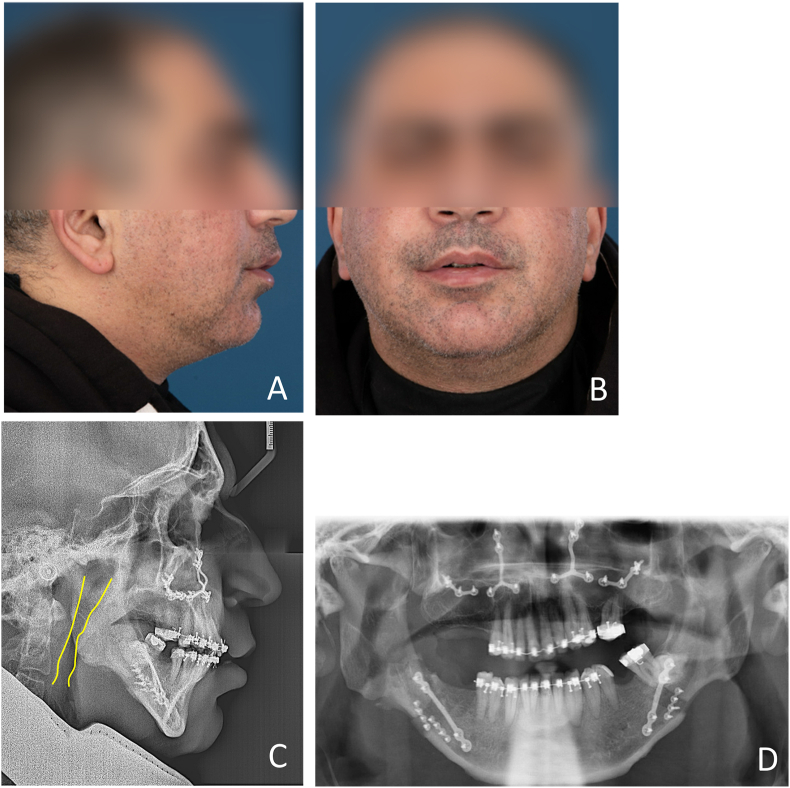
After maxillomandibular advancement surgery. A, profile view of the extra-oral photograph; B, frontal view of the extra-oral photograph; C, lateral cephalogram; D, panoramic radiograph.
